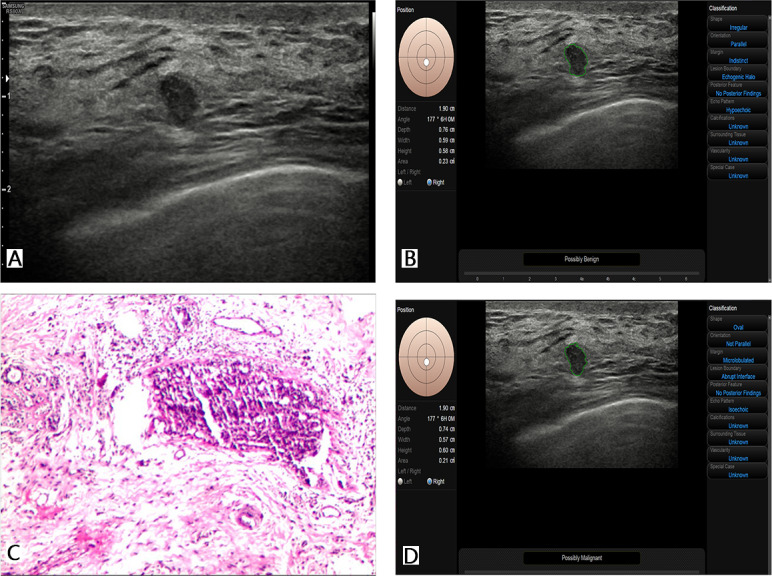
Case 1: a woman aged 27 years was found to have a left breast mass during routine physical examination. Ultrasound revealed a hypoechoic lesion measuring approximately 0.60 × 0.57 cm in the glandular layer beneath the left nipple, adjacent to the areola. The lesion exhibited relatively circumscribed margins and a slightly irregular shape, with a nonparallel orientation. No posterior acoustic attenuation or architectural distortion of the surrounding tissue was observed (A). The initial S-Detect assessment suggested a benign lesion (B), whereas the repeated assessment indicated possible malignancy, demonstrating discordant results (D). Postoperative pathology confirmed usual ductal epithelial hyperplasia with inflammatory cell infiltration (C).
